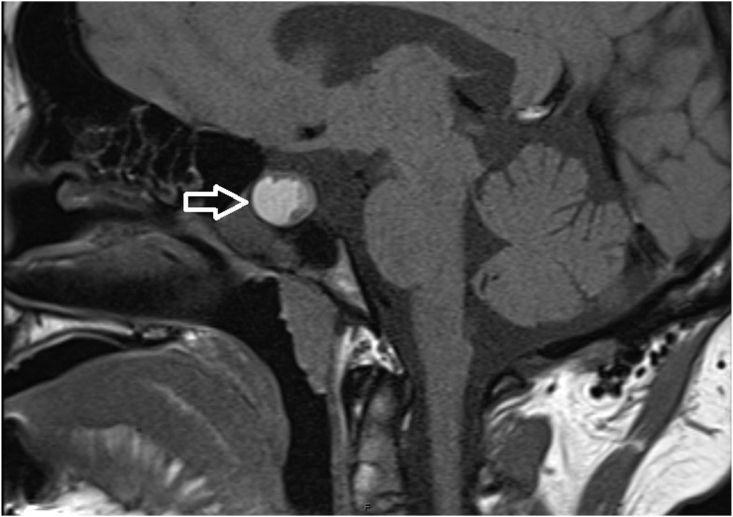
Magnetic resonance imaging of pituitary notable for a 1.4 cm well-circumscribed, hemorrhagic sellar lesion (white arrow).
